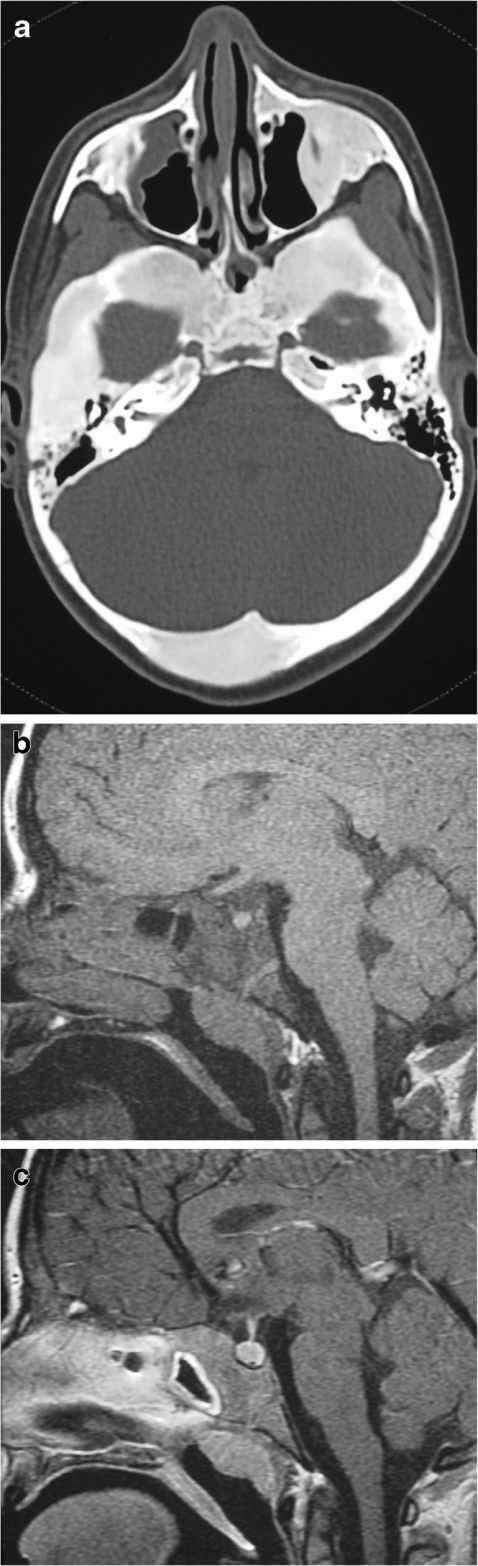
Fibrous dysplasia in a 5-year-old girl with McCune Albright syndrome. Axial CT (a) shows multiple expansile lesions involving the skull which have a “ground glass” appearance representing sites of polyostotic fibrous dysplasia. Sagittal T1WI shows expansion of the clivus which has diffuse low signal (b) with corresponding diffuse enhancement on sagittal post-contrast fat-suppressed T1WI (c)
